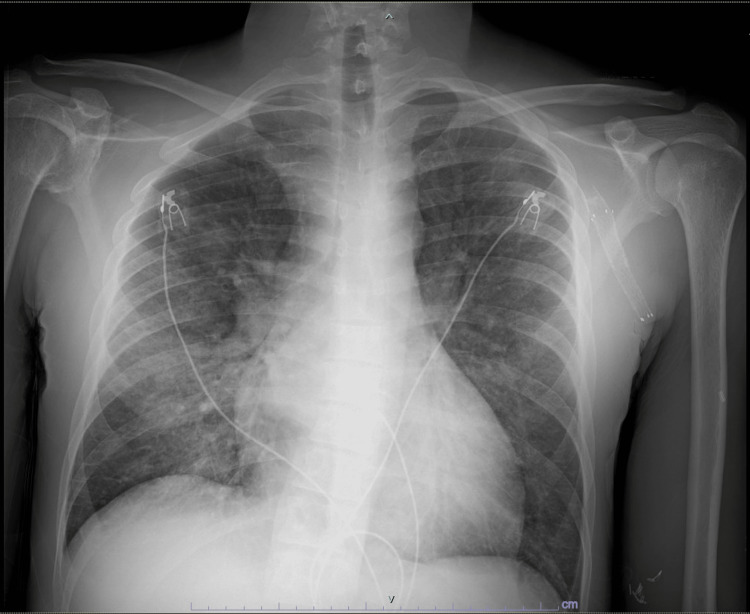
Chest radiograph (frontal view) demonstrating a right perihilar airspace opacity concerning for infection.
